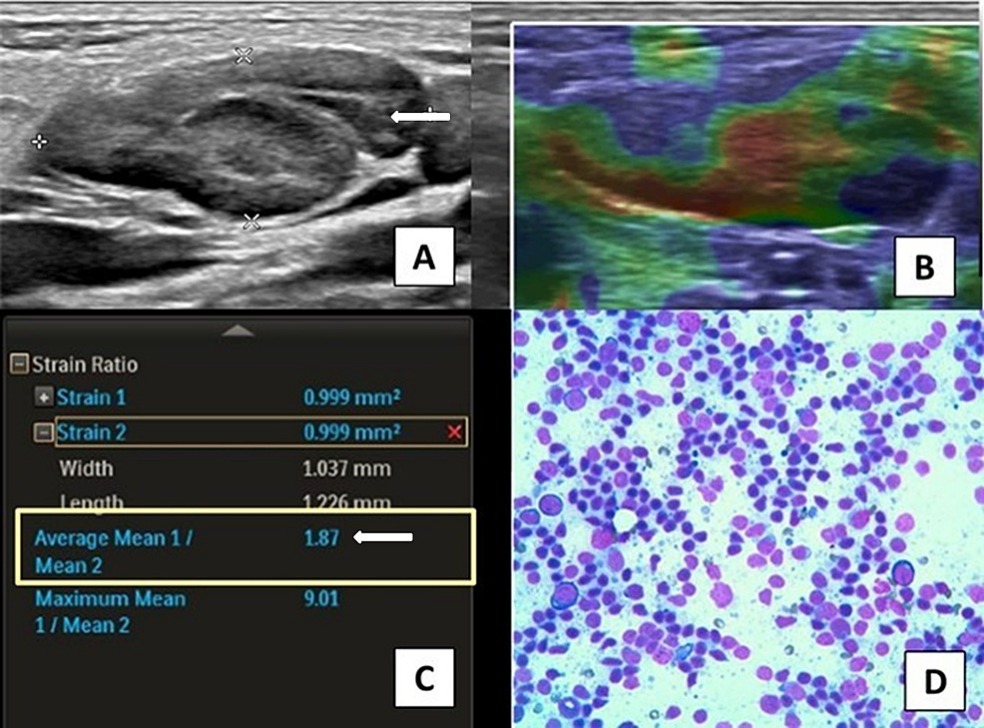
Four-year-old male patient presented with cervical lymphadenopathy (A) On USG, lymph nodes are oval in shape with preserved fatty hilum (arrow). (B and C) On elastography, the lymph node has elastography pattern 2 and SI of 1.87 (arrow). USG and elastography findings are suggestive of benign etiology. (D) FNAC of the lymph node shows reactive cervical lymphadenitis. USG: ultrasonography; SI: strain index; FNAC: fine-needle aspiration cytology
